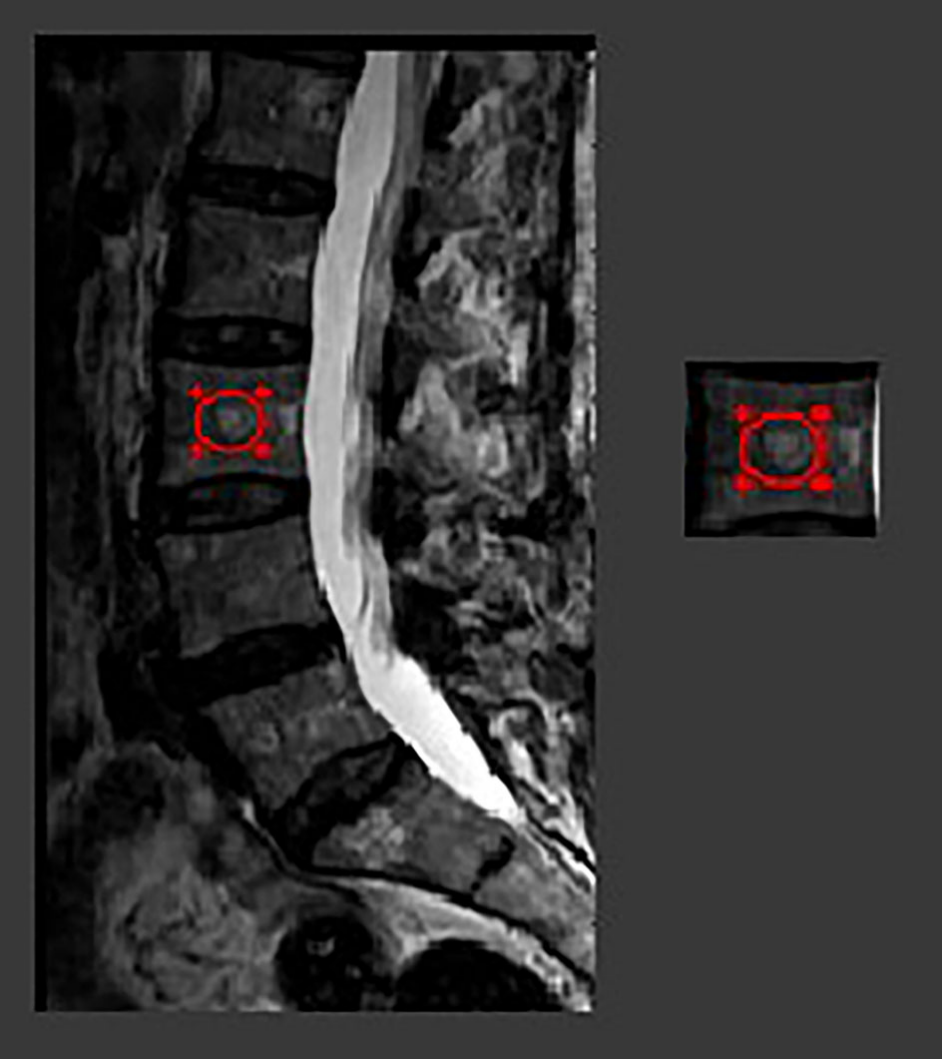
Image demonstrating the region of interest positioning in the central region of L3 vertebra on the sagittal T2-weighted fast spin echo MRI sequence.
